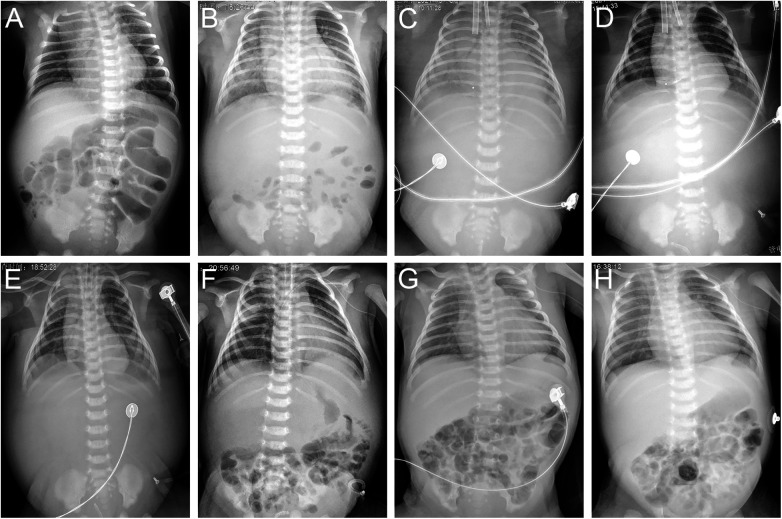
Chest and abdominal radiographs on DOH 1 (A), DOH 4 (B), DOH 6 (C), DOH 8 (D), DOH 10 (E), DOH 12 (F), DOH 19 (G) and DOH 21 (H). Increasingly intensified pulmonary edema, disappeared intestinal tube aeration and accumulated ascites (A–C), gradually subsided pulmonary edema, recovered intestinal tube aeration and absorbed ascites (D–F), enlargement of the heart shadow, relatively clear lung field and well-inflated intestinal tube (G,H). DOH, day of hospitalization.
